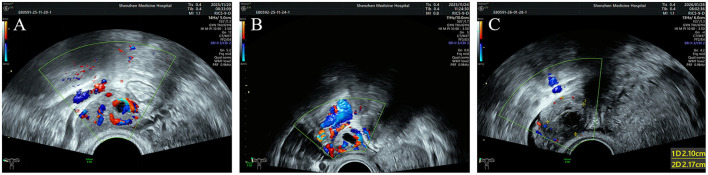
Transvaginal ultrasound images before, during, and after treatment. (A) 2025-11-20 First ultrasound after admission: a mixed echo was seen next to the right ovary, about 13 × 13 mm in size. (B) 2025-11-24 Ultrasound after the first MTX treatment: a mixed echo was seen next to the right ovary, about 16 × 13 mm in size. (C) 2026-01-28 Ultrasound when HCG turned negative: a mixed echo was seen next to the right ovary, about 22 × 21 × 22 mm in size.
